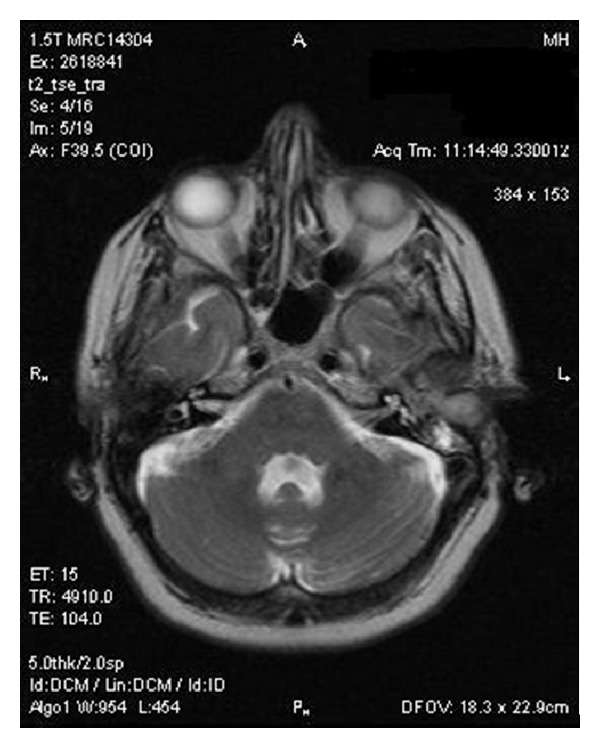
Axial T2 weighted MRI image demonstrating the left sided tumour mass.
